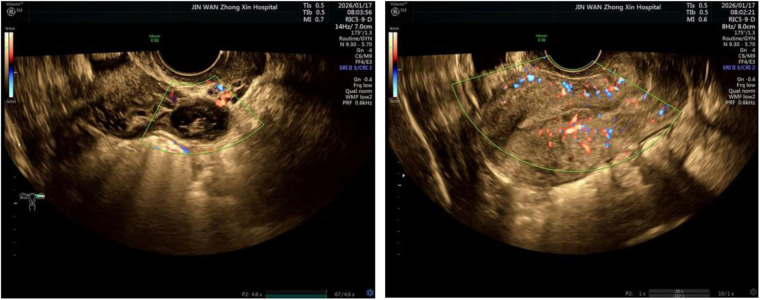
Transvaginal ultrasound on day 14 of treatment (January 17, 2026). The ectopic mass decreased to 20 × 18 mm with peripheral blood flow signals reduced to punctate and linear patterns, indicating the absorption phase.
